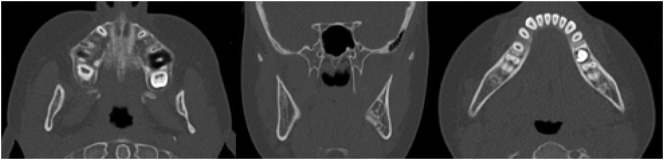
CT scan performed 18 months after starting triptorelin treatment showed complete restoration of the jaw anatomy and total regression of the osteomyelitis.
